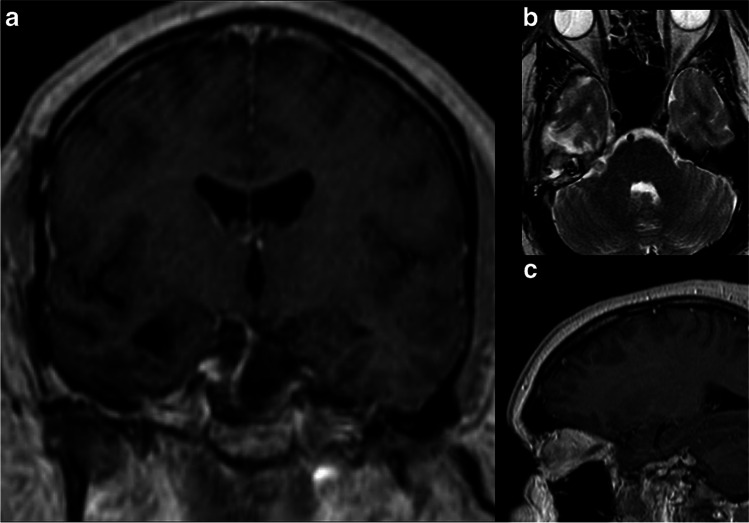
a Postoperative T1-weighted coronal MRI cut after gadolinium injection, showing a complete resection of the middle fossa component. b Postoperative T2-weighted MRI cut showing mild edema in the basal aspect of the temporal lobe, as well as a small tumor remnant in the cerebellopontine angle. c Postoperative T1-weighted sagittal MRI cut after gadolinium injection, showing a complete resection of the middle fossa and intrameatal components, and a small tumor remnant in the posterior fossa
